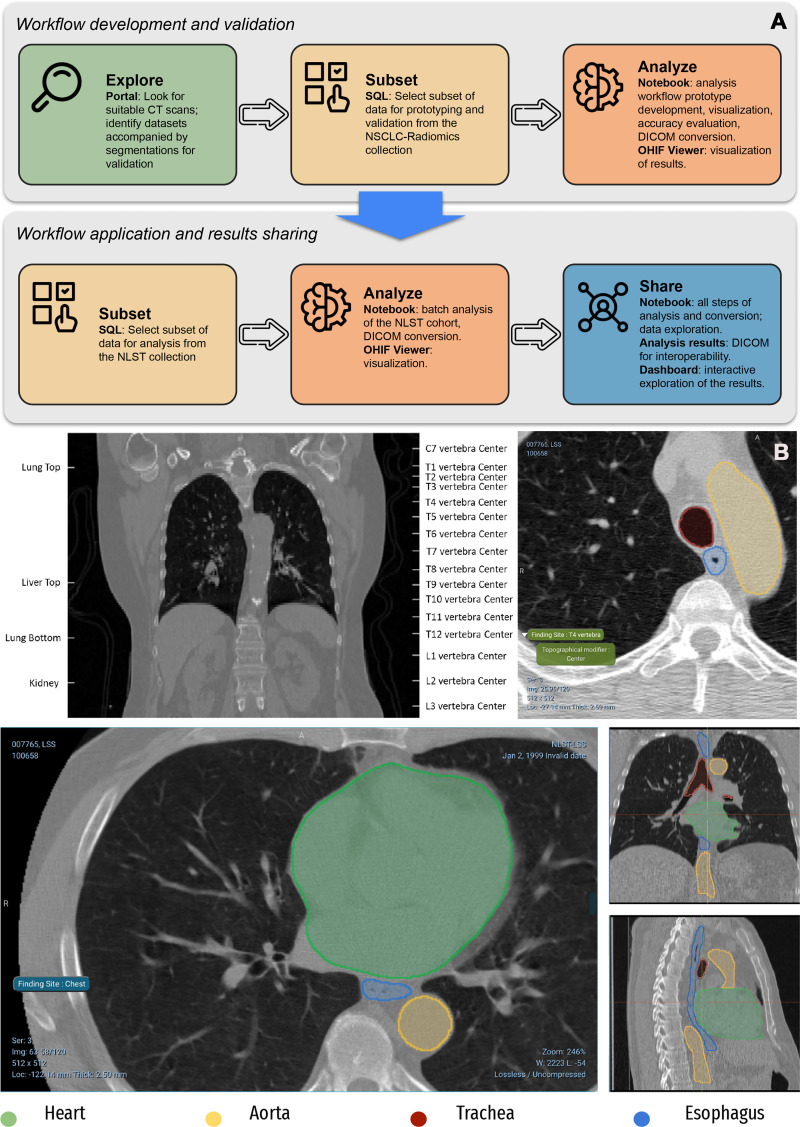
AI-generated annotations of CT images using BodyPartRegression (43) and the nnU-Net Task055 SegTHOR
